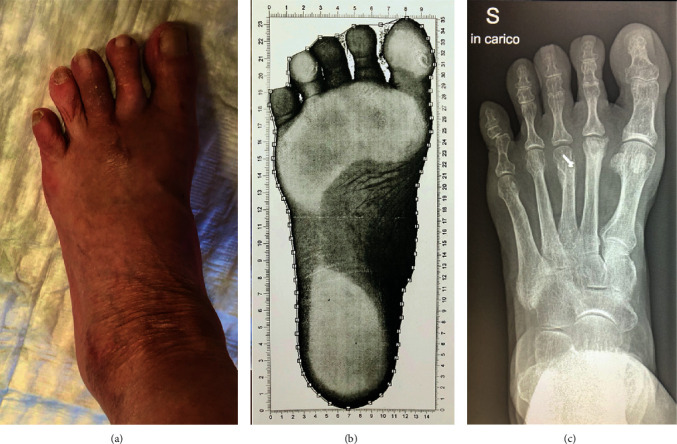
Results at the 3-month follow-up after removing the K-wire, with (a) photograph while weight bearing, (b) walking analysis, and (c) plane X-ray.
